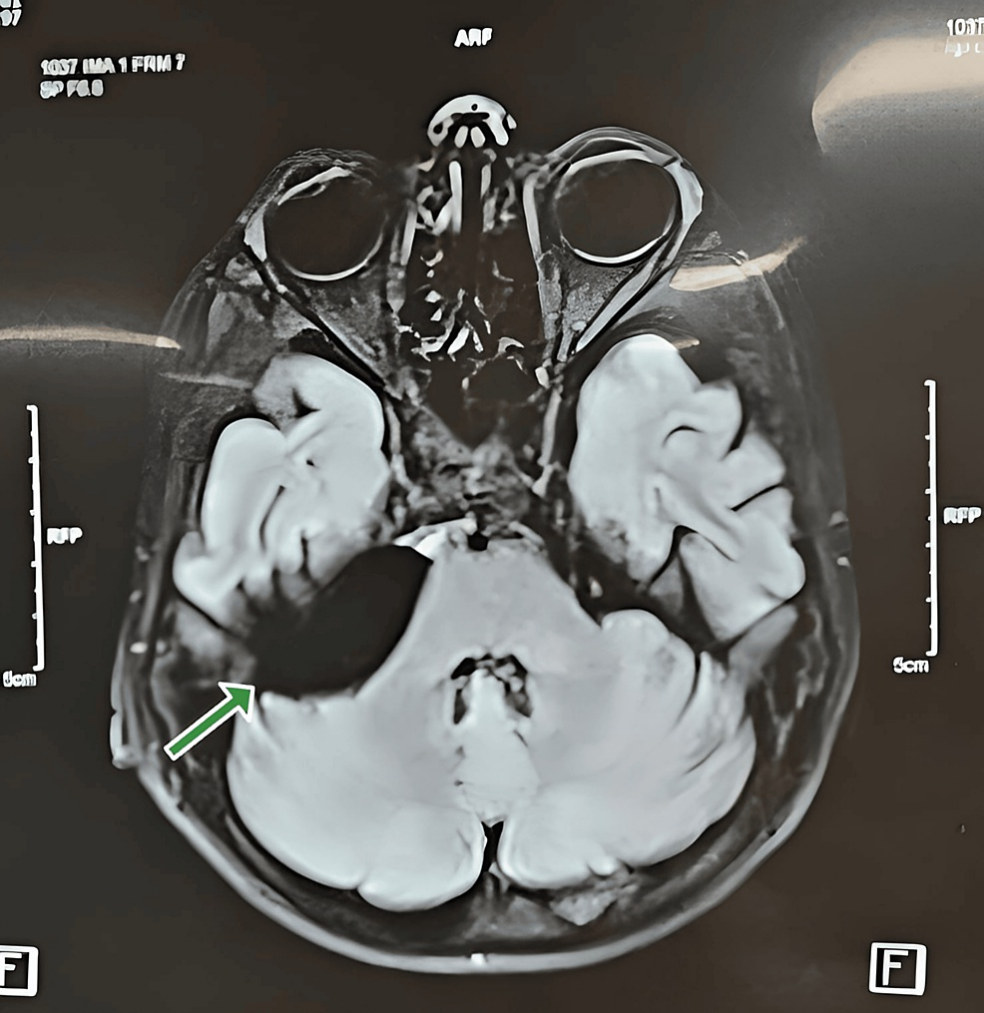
Magnetic resonance imaging through the posterior fossa demonstrated a large right-sided extra-axial cerebrospinal fluid intensity mass lesion. There is a significant mass effect on the adjacent cerebellar tissue.
